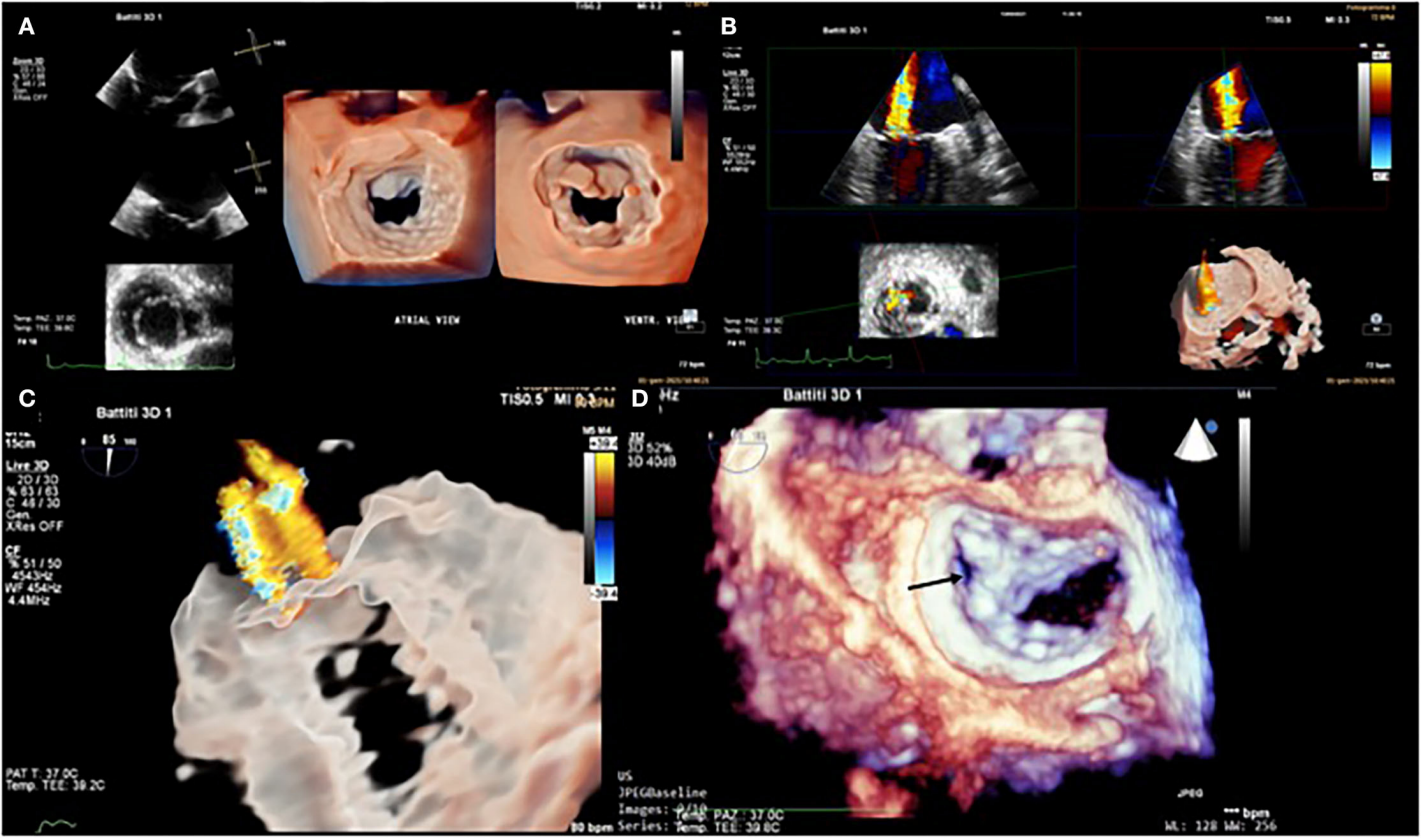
Transesophageal echocardiogram. 3D mitral valve reconstruction with atrial view (or “surgeon's view”) and ventricular view sergi (A). Severe mitral regurgitation (MR) was documented with 3D color Doppler acquisition (B). 3D Glass image of MR (C). 3D mitral valve reconstruction (atrial view) after Mitra-Clip system placement in the lateral paracommissural region (black arrow) (D).
